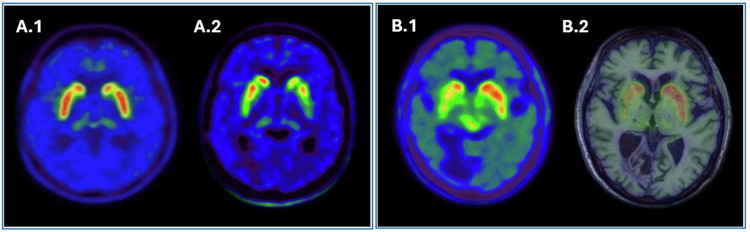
A [^18^F]FDOPA PET image of a healthy patient (A.1) and a patient with PD (A.2). B Pitfalls and artefacts in presynaptic dopaminergic imaging. [^18^F]FDOPA PET scan (B.1) of a patient with suspected Parkinson’s disease showing a decrease in the right putamen. However, the fusion PET/MRI image (B.2) shows that the decreases are due to the artefact of spaces surrounding the walls of vessels within the brain parenchyma observed on MRI
